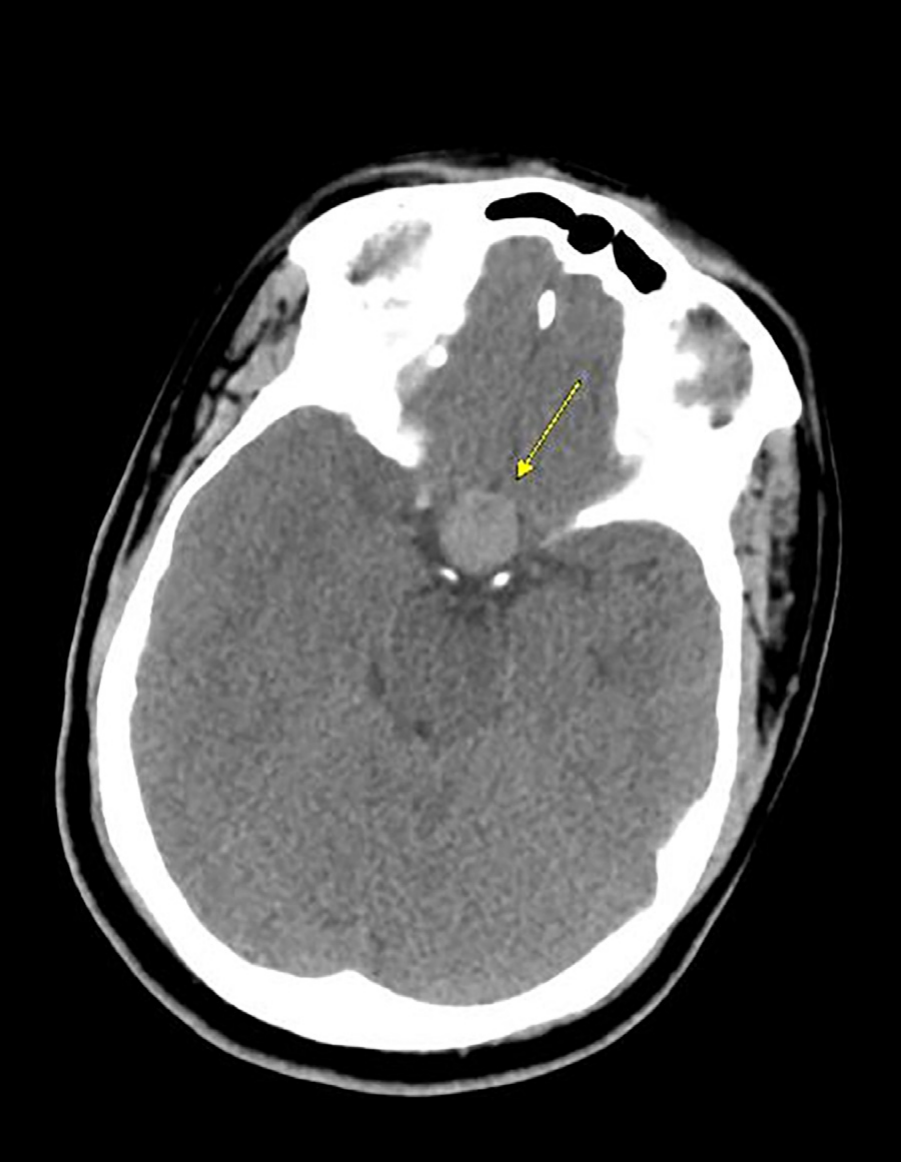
Initial imaging with Axial Noncontrast CT head taken June 2021 showing a heterogeneously hyperdense lesion (yellow arrow) in the suprasellar cistern with central hypodensity that appears to be extending from the sella turcica and measures approximately 1.8 × 1.7 × 3.0 cm, causing mass effect upon the optic chiasm. Differential at this stage includes pituitary macroadenoma, or less likely meningioma, or less likely aneurysm.
